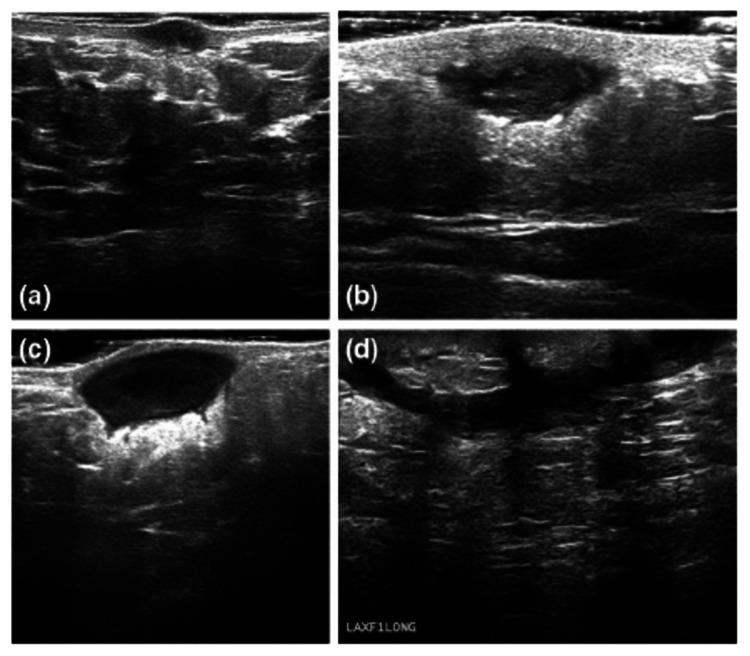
Ultrasound showing the types of HS lesions - (a) superficial nodule, (b) deep nodule, (c) abscess, and (d) tunneling. HS: hidradenitis suppurativa
